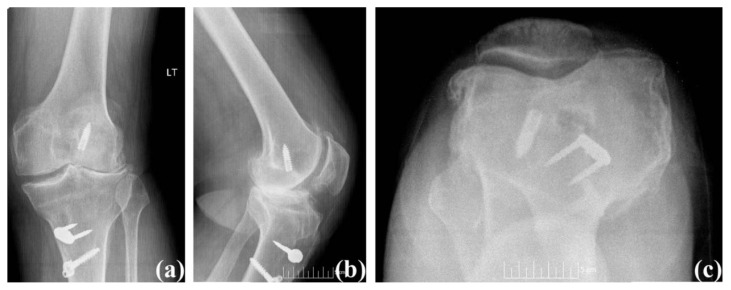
Case #3 pre-operative radiograph: (a) anterior posterior view, (b) lateral view, and (c) sunrise view.
